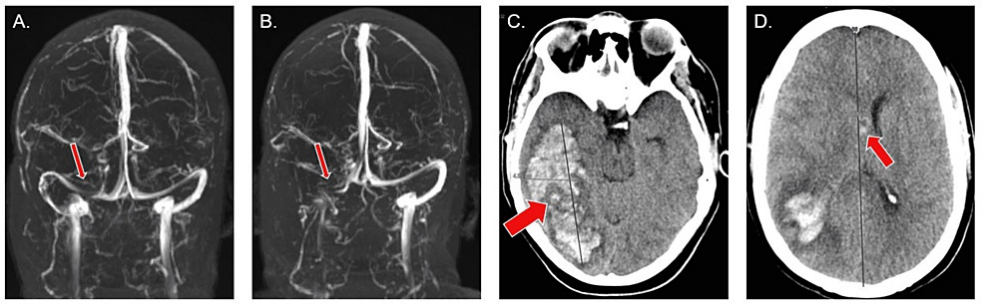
Selected relevant neuroimaging. (A) MRV on hospital day four, revealing a non-obstructing thrombus at the junction of the right transverse and sigmoid sinuses (see arrow). (B) MRV on hospital day 10 with interval progression of the thrombus in the right transverse and sigmoid sinuses without flow (see arrow). (C) CT without contrast, showing a large occipital and temporal lobe intraparenchymal hematoma. (D) The different axial cut of the same CT without contrast shows 7 mm of midline shift (arrow) and intraventricular extension of the hematoma (arrow). MRV: magnetic resonance venogram; CT: computed tomography; mm: millimeters.
